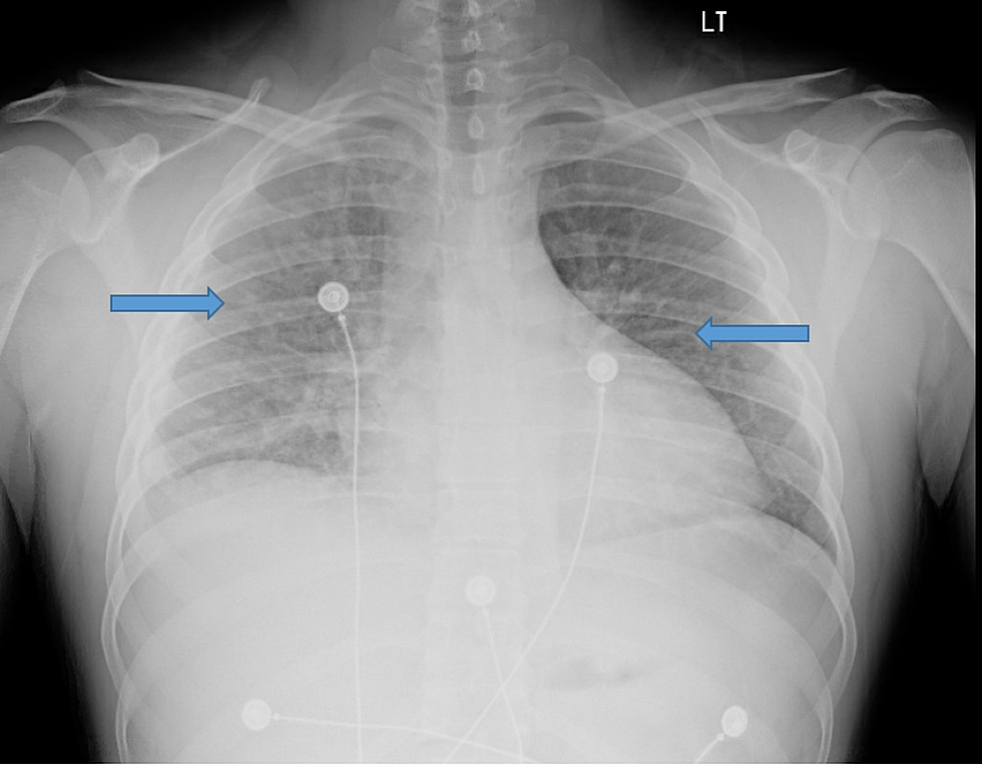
Chest x-ray, AP view, showing increased interstitial lung markings bilaterally. Anteroposterior (AP)
